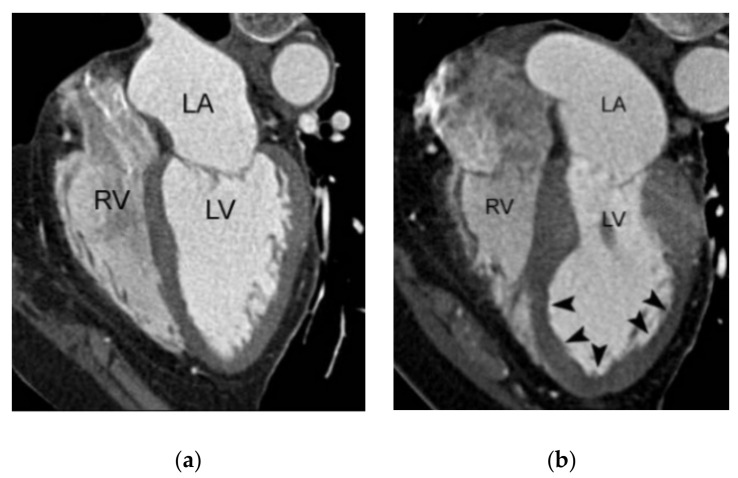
A case of stress-induced cardiomyopathy in a 65-year-old female patient identified on coronary CTA. Typical apical ballooning (arrowheads in (b)) is noted on a systolic four-chamber image in comparison to a diastolic image (a). There was no obstructive coronary artery stenosis on coronary CTA or invasive coronary angiography (not shown).
